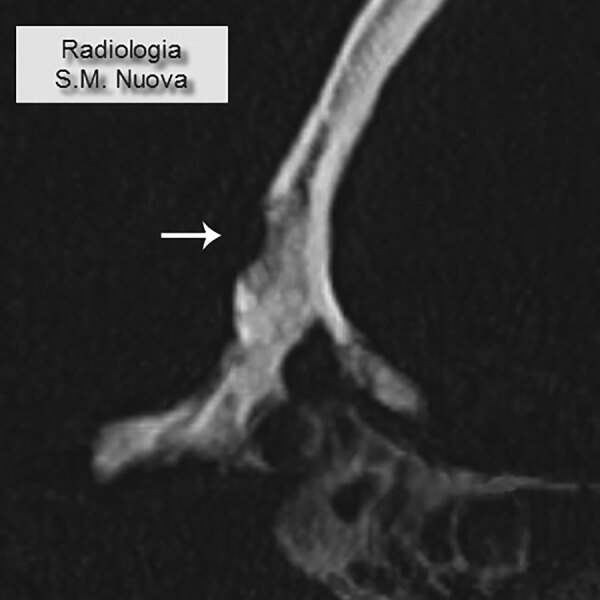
Computed tomography scan of frontal bone with an osteolytic lesion with sclerotic walls (circumvallate cavitations). (Archive of the Division of Paleopathology. University of Pisa.)
